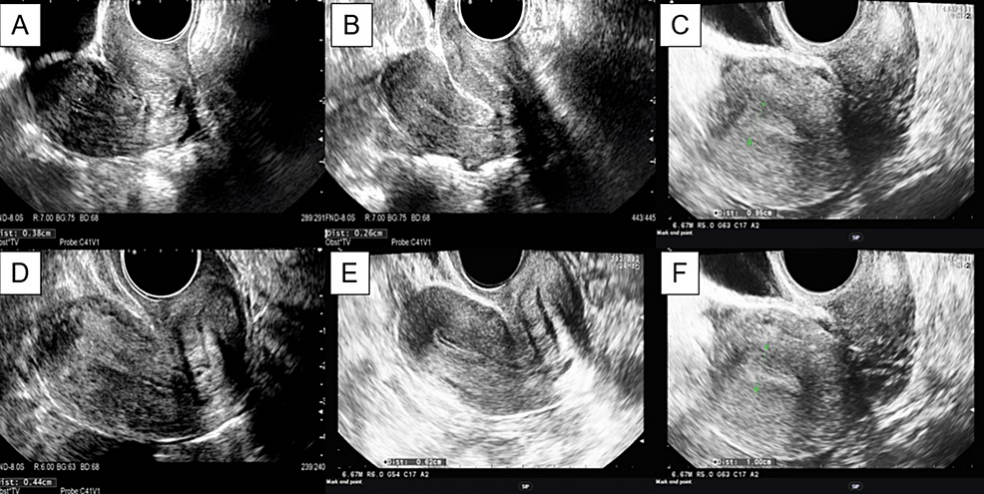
Endometrial thickness measured with transvaginal ultrasound in patient 3 (A) Thin endometrium (3.8 mm) in the secretory phase of the menstrual cycle before trial registration. (B) Endometrium directly after menstruation (2.6 mm) before ADRC treatment. (C) Uterine cavity filled with ADRC suspension with hyaluronic acid and estradiol-17β (9.5 mm). (D) Slightly thickened endometrium (4.4 mm) on the second day after intrauterine ADRC infusion. (E) Endometrium with a multilayered appearance in the late proliferative phase (6.2 mm). (F) Marked improvement of endometrial thickness in secretory phase after first ADRC infusion (10 mm). ADRC: adipose tissue-derived regenerative cell
